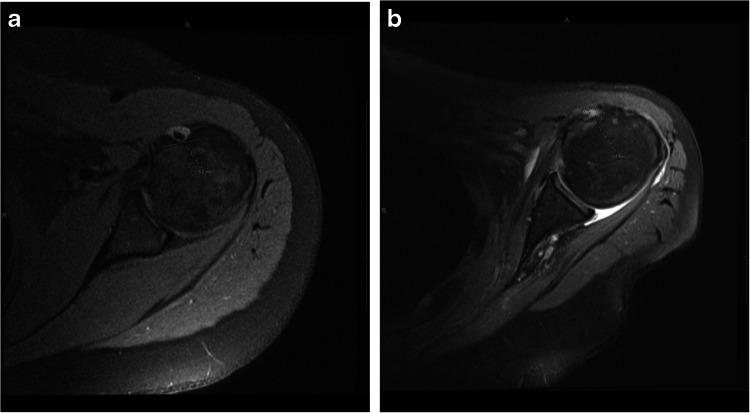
Axial MR images of normal LHBT and chronically torn LHBT. A A 53-year-old woman with an intact long head biceps tendon in the bicipital groove, with groove depth measuring 5.0 mm. B A 59-year-old woman with a complete tear of the long head biceps tendon, with shallowing of the bicipital groove, demonstrating a groove depth of 3.1 mm
